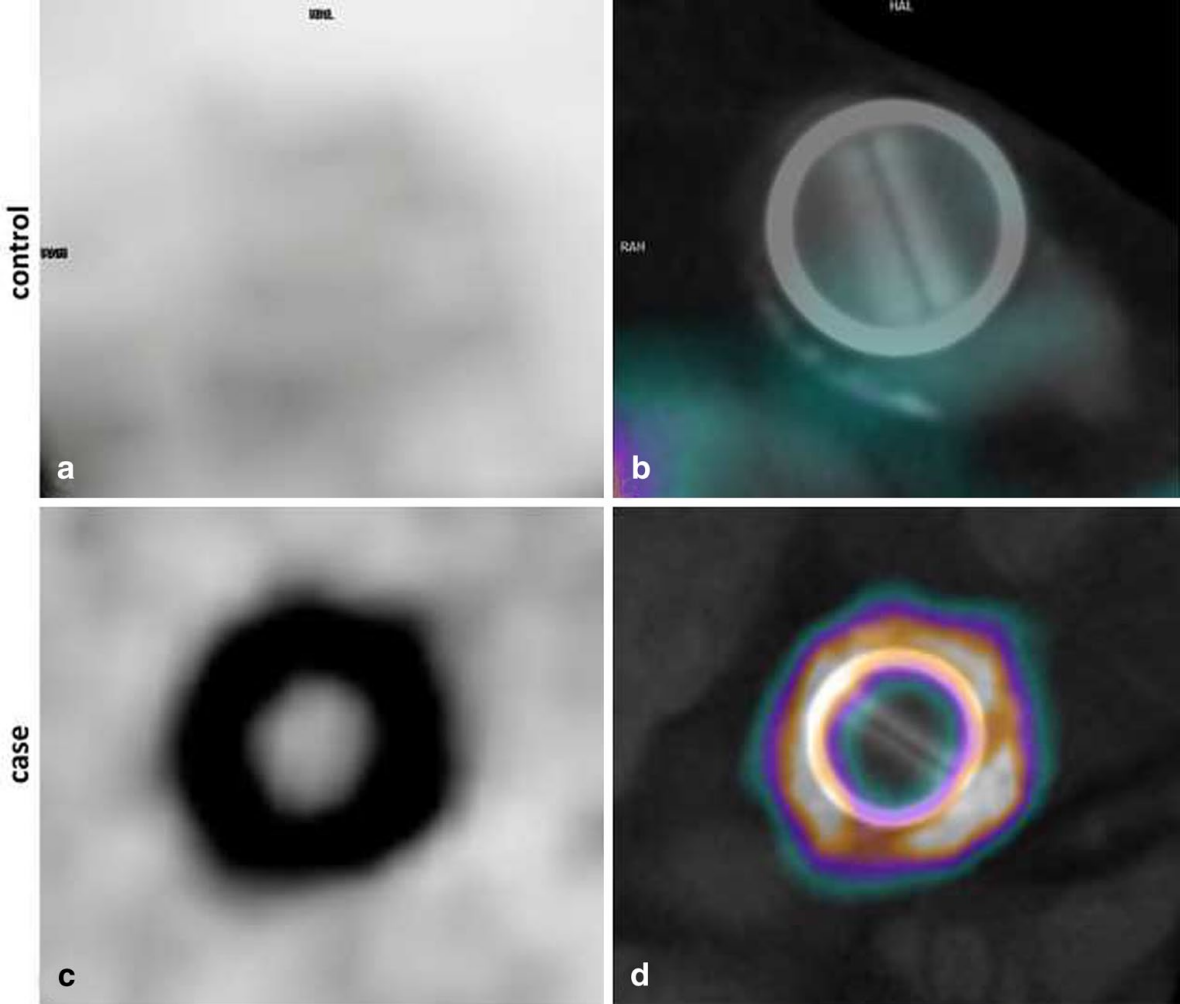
Case *versus* Control. Panel a and b: Aortic bileaflet mechanical PHV implanted 16 months previously, multiple blood cultures were positive for Proprionii bacterium. TTE and TEE did not show abnormalities and modified Duke criteria were not fulfilled. FDG-PET (a) showed severe FDG uptake. After fusion with MDCT, the FDG uptake was detected around the PHV ring (b), suggestive of widespread periannular extension of endocarditis around the whole PHV, which was confirmed at subsequent surgical inspection and pathological examination. Panel c and d: Aortic bileaflet St. Jude prosthesis in the chronic postoperative phase without suspected endocarditis. Metabolic imaging by FDG-PET on the level of the PHV did not show uptake. Re-printed with permission [20]
